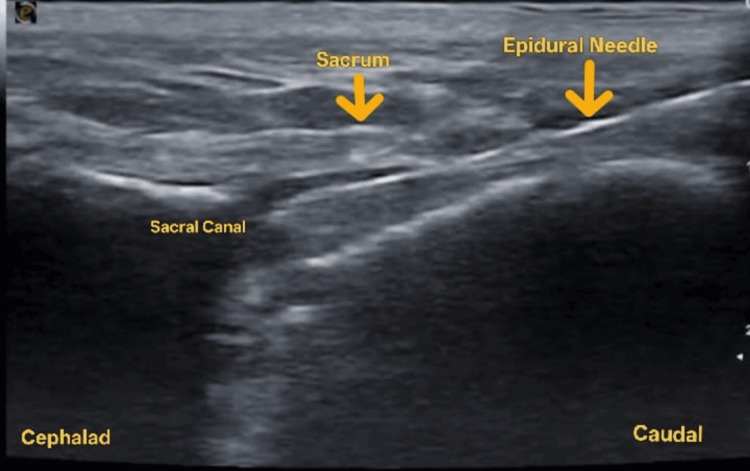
Ultrasound image showing longitudinal view of sacral hiatus
